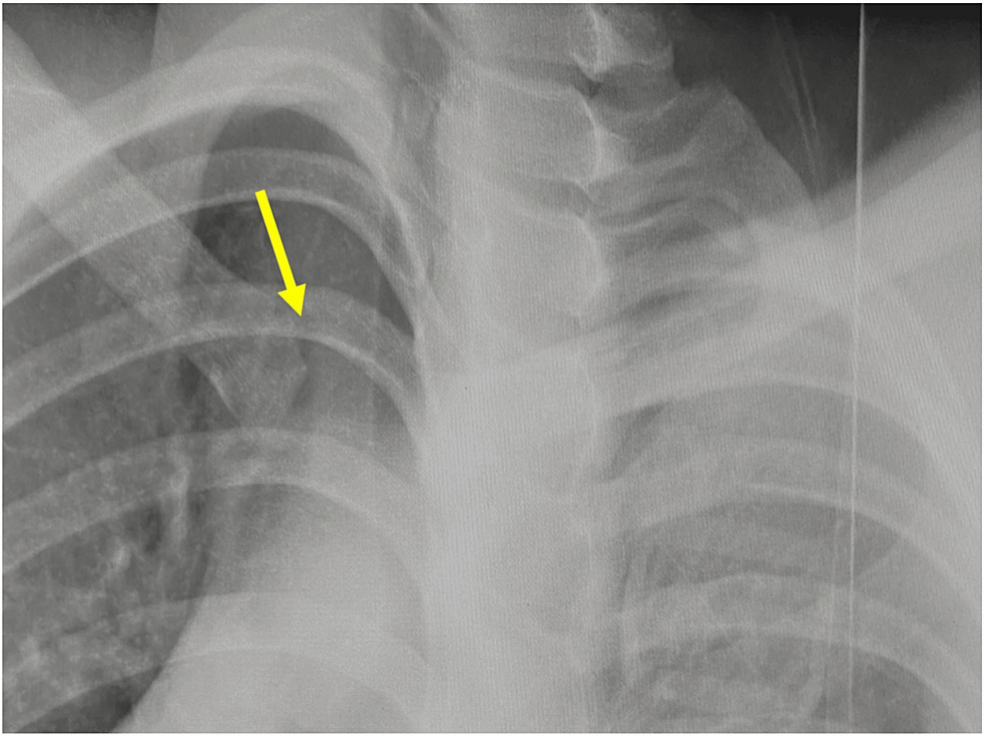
On oblique radiography, the right clavicle was slightly displaced distally (→).
